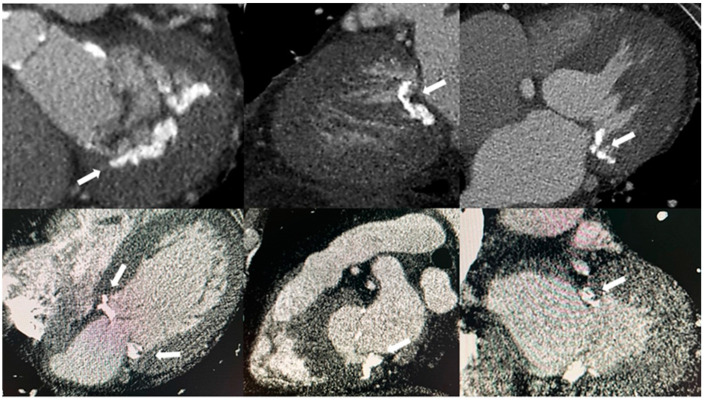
The appearance of mitral annular calcification on computed tomography (white arrows).
